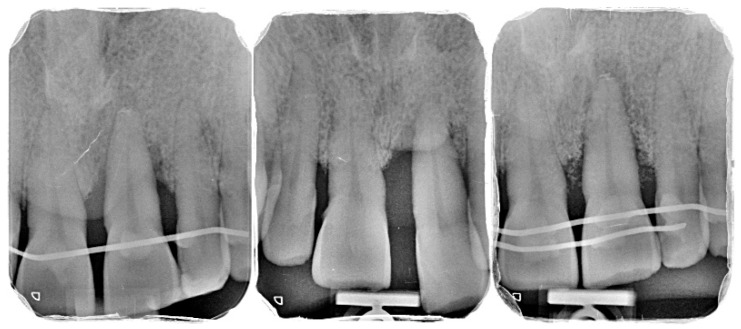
Standardized periapical radiograph, baseline and surgical day.
